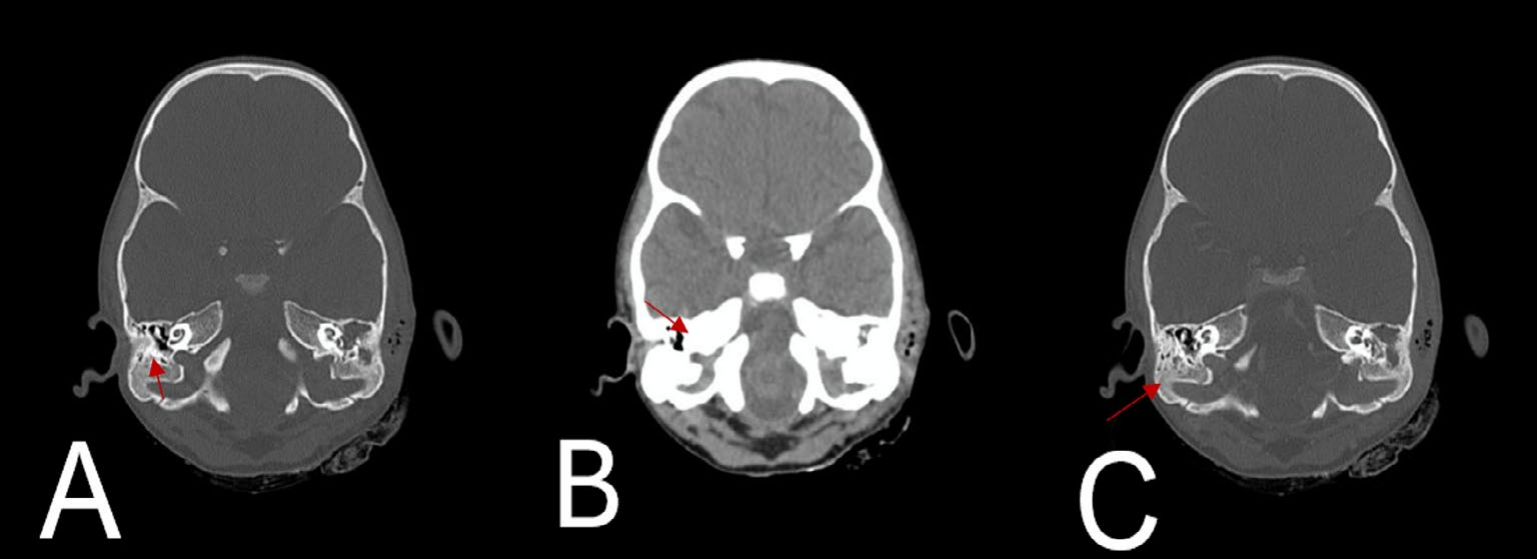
(A) Contrast‐enhanced CT scan of the brain showing the right mastoid air cells (arrow) show sclerosis and partial opacification consistent with chronic mastoiditis. (B) A slightly anterior section demonstrates soft‐tissue density opacification within the right middle ear cavity (arrow), involving the epitympanum and mesotympanum, indicating otitis media with effusion or granulation tissue formation. (C) A more posterior image shows residual fluid density within the right mastoid tip (arrow) without bony destruction or intracranial extension. The left mastoid and middle ear are well aerated in all sections.
